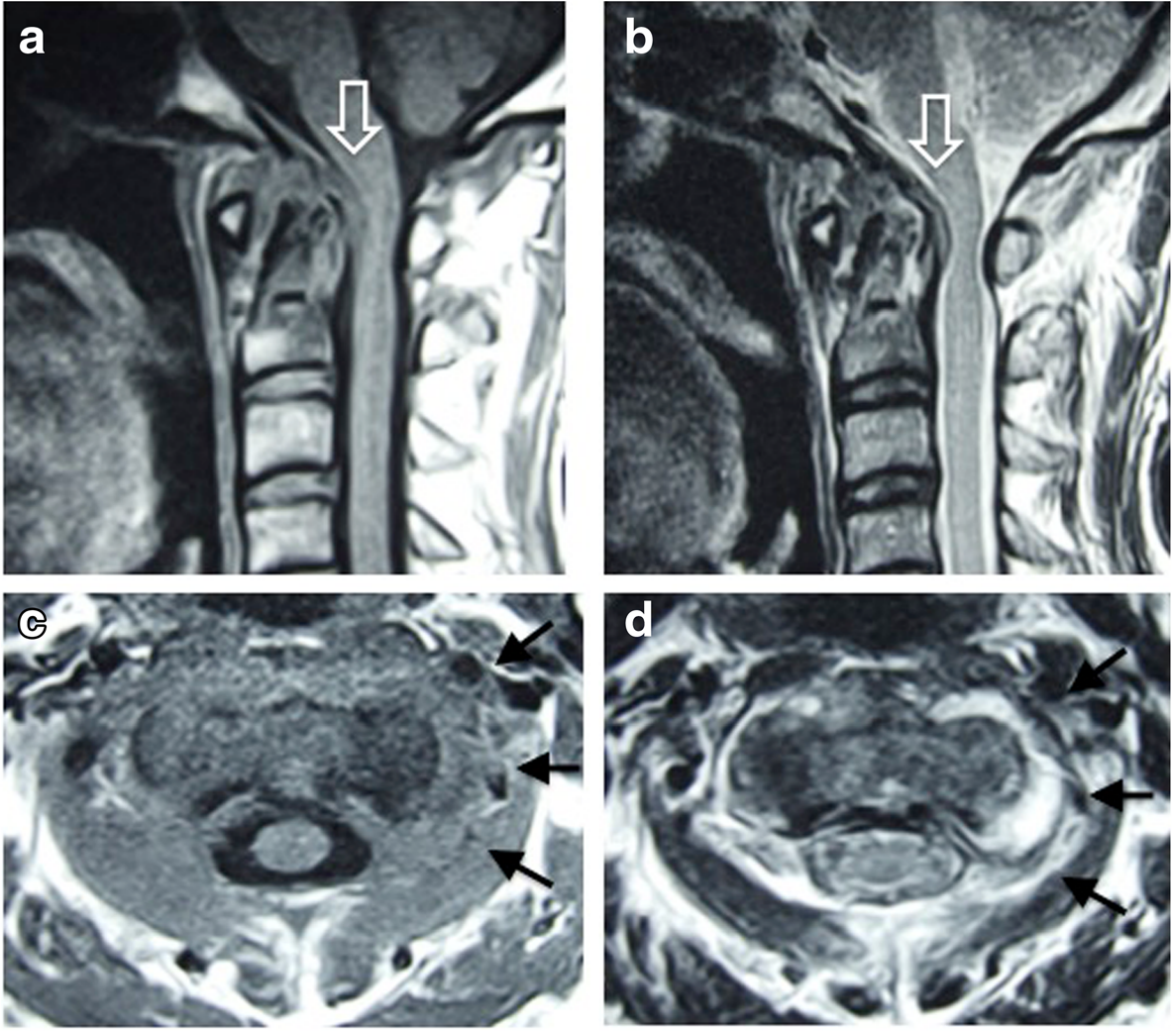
Magnetic resonance imaging on admission. Sagittal imaging (a, b) shows cord compression due to a pseudotumor around the dens (*open arrow*). Axial imaging shows heterogeneously low signal intensity around the left lateral atlantoaxial joint on T1-weighted imaging (c, *arrows*) and high signal intensity on T2-weighted imaging (d, *arrows*)
